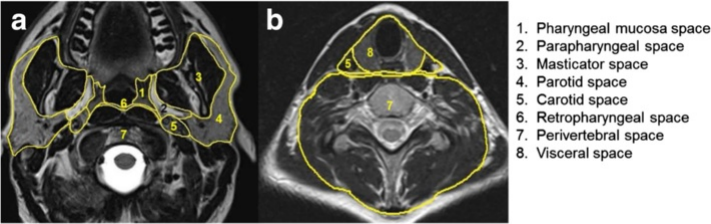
Axial T2-weighted images showing the deep spaces of the suprahyoid neck at the level of the oropharynx a and the infrahyoid neck at the level of the thyroid b which are demarcated by the deep fascia of the neck. The retropharyngeal space is a potential space between the perivertebral space and the pharyngeal mucosa space above and the visceral space below the level of the hyoid bone
